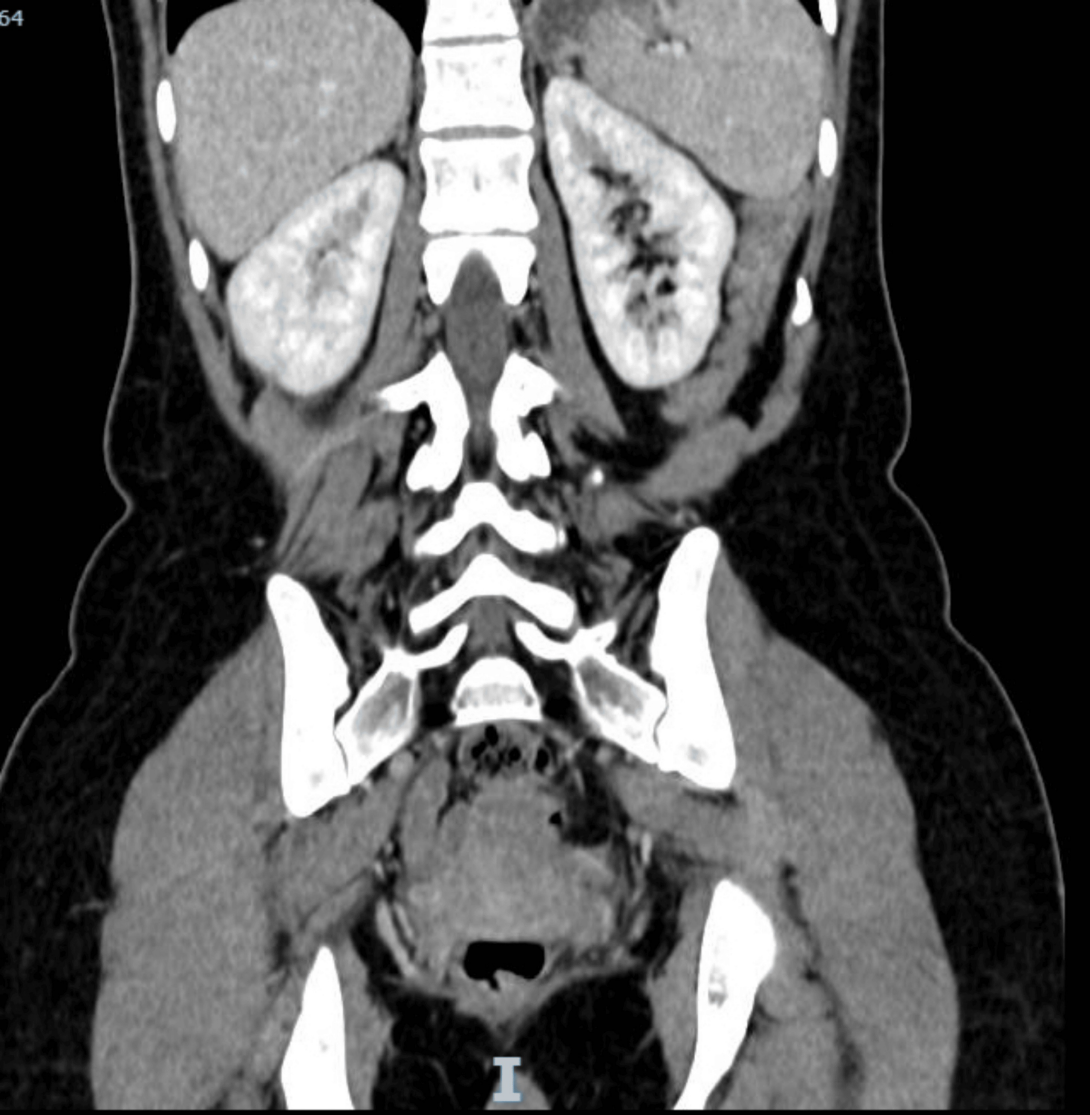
Postoperative CT scan confirming removal of adrenal tumor
